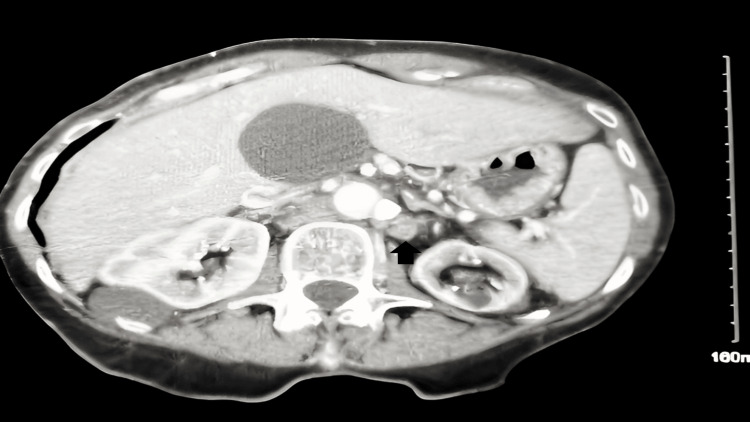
CT abdomen pelvis with IV contrast reveals peri-aortic density on the left that could represent occluded vessel versus adenopathy
